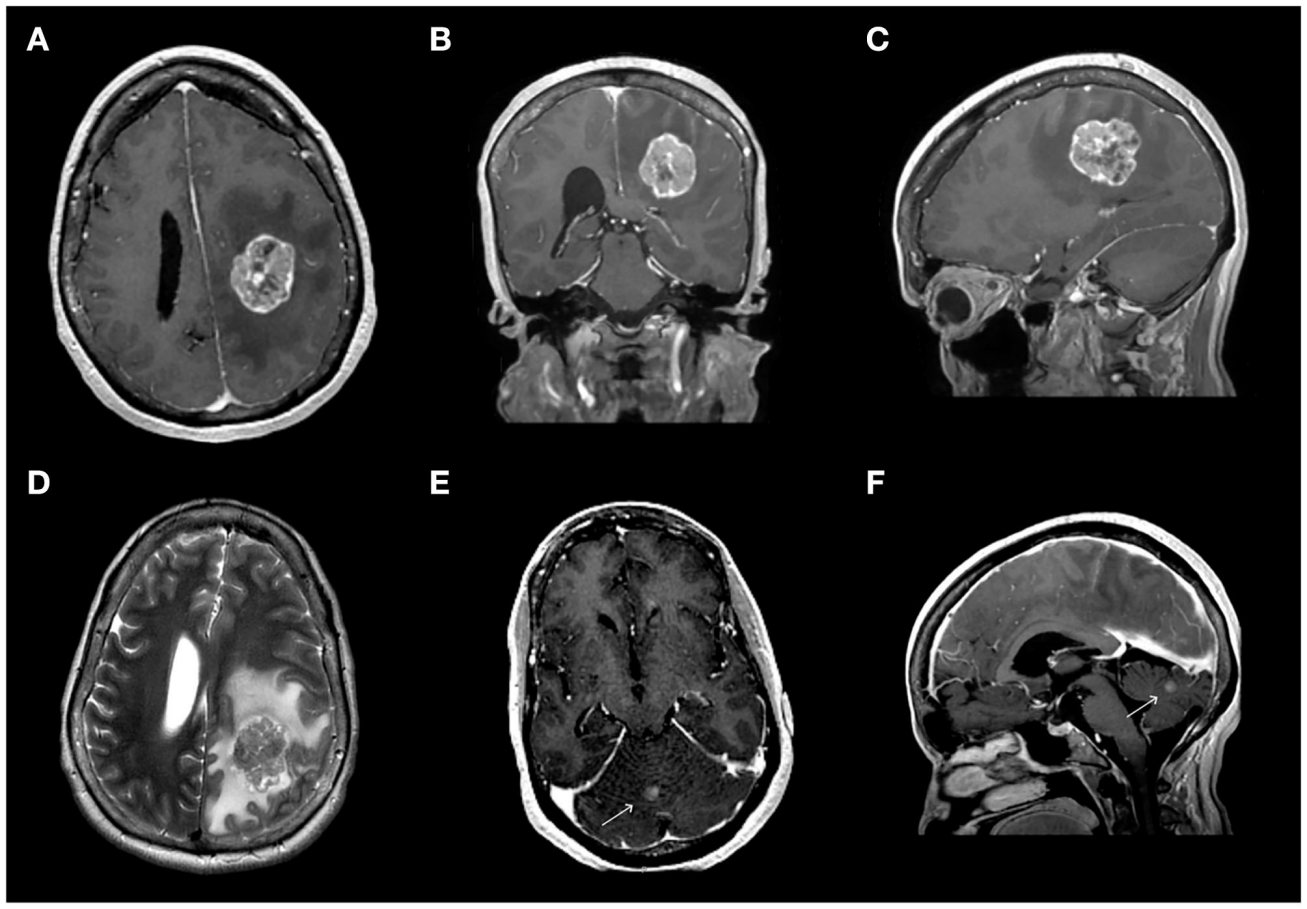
Pre-operative magnetic resonance imaging (MRI) revealed a dominant 3.7 × 2.7 × 3.7 cm left frontoparietal mass associated with diffuse edema (A–D) and a 6 mm lesion in the cerebellar vermis (E,F). (A–C) Contrast-enhanced T1-weighted MR sequence showing the dominant frontoparietal lesion in the axial (A), coronal (B), and sagittal (C) views. (D) T2-weighted image of the dominant lesion. (E,F) Contrast-enhanced T1-weighted MR sequence showing the lesion in the cerebellar vermis in axial (E) and sagittal (F) views.
